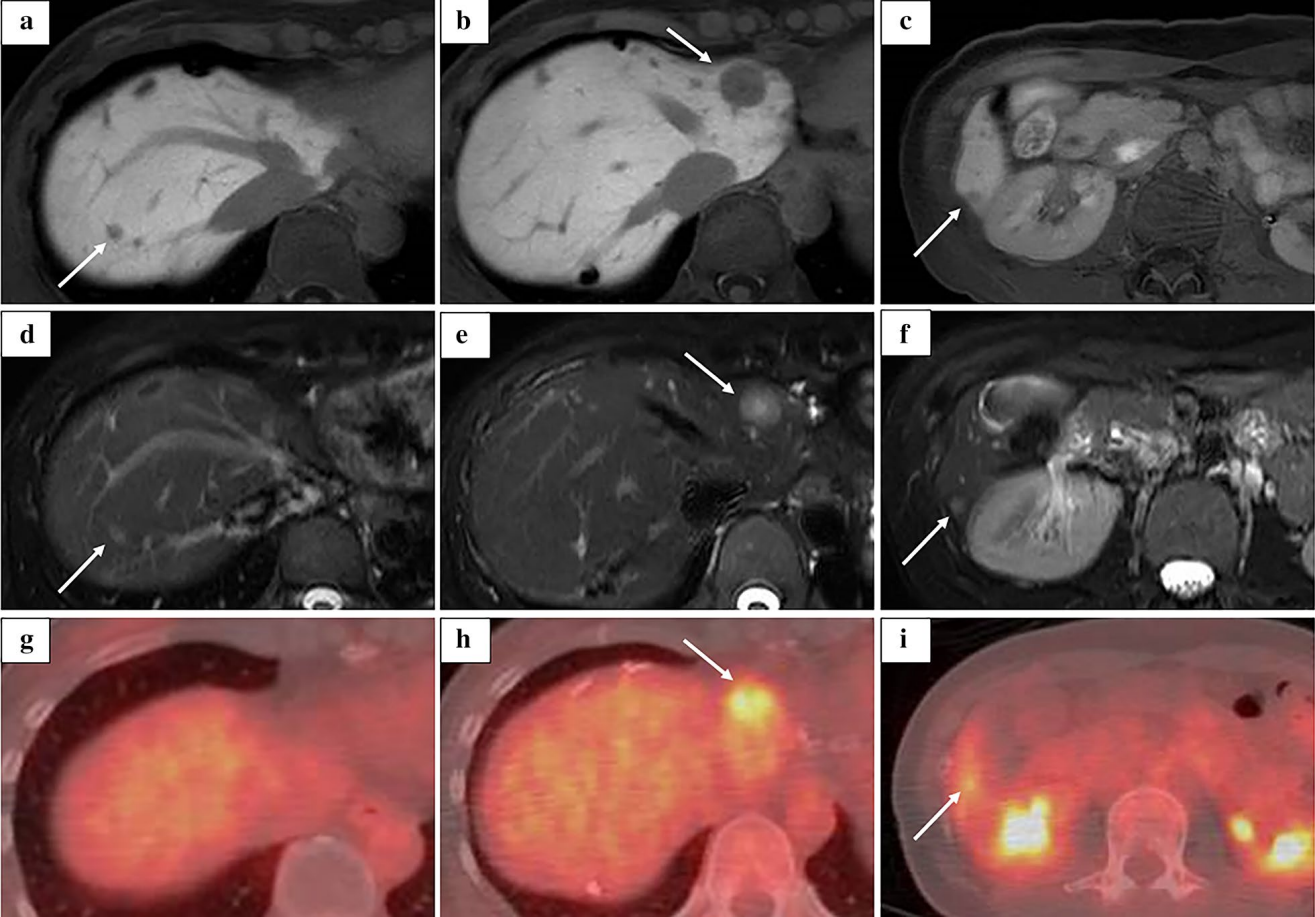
a–f Follow-up Gd–EOB–DTPA enhanced MRI and T2-weighted MRI at 15 months after the primary surgery revealed a 18-mm tumor in the medial section (S4) (b, e, arrow), a 7-mm tumor in the posterior inferior segment (S6) (c, f, arrow), and a 4-mm tumor in the anterior superior segment (S8) (a and d, arrow). g–i Enhanced FDG-PET showed uptake of FDG in the tumor in S4 and S6 (h, i)
